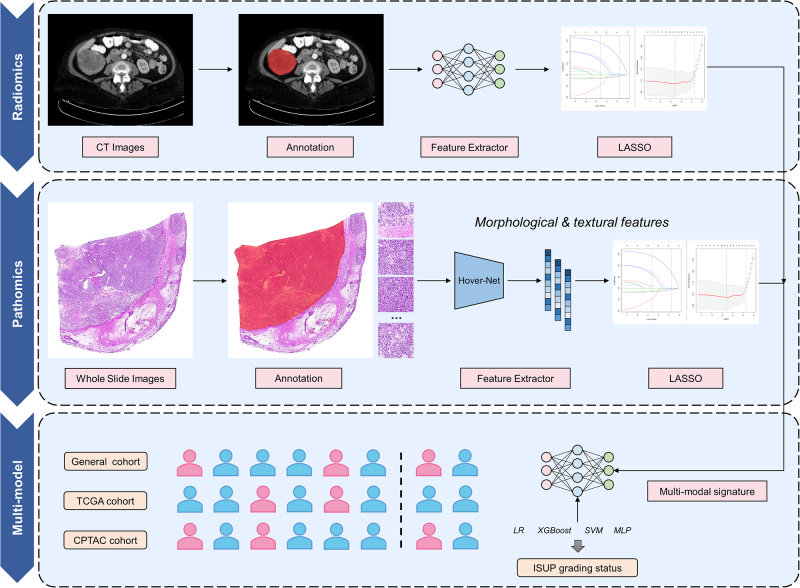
Study design and analysis process of this study. CPTAC, Clinical Proteomic Tumor Analysis Consortium; CT, computed tomography; ISUP, International Society of Urological Pathology; LASSO, least absolute shrinkage and selection operator; TCGA, The Cancer Genome Atlas.
